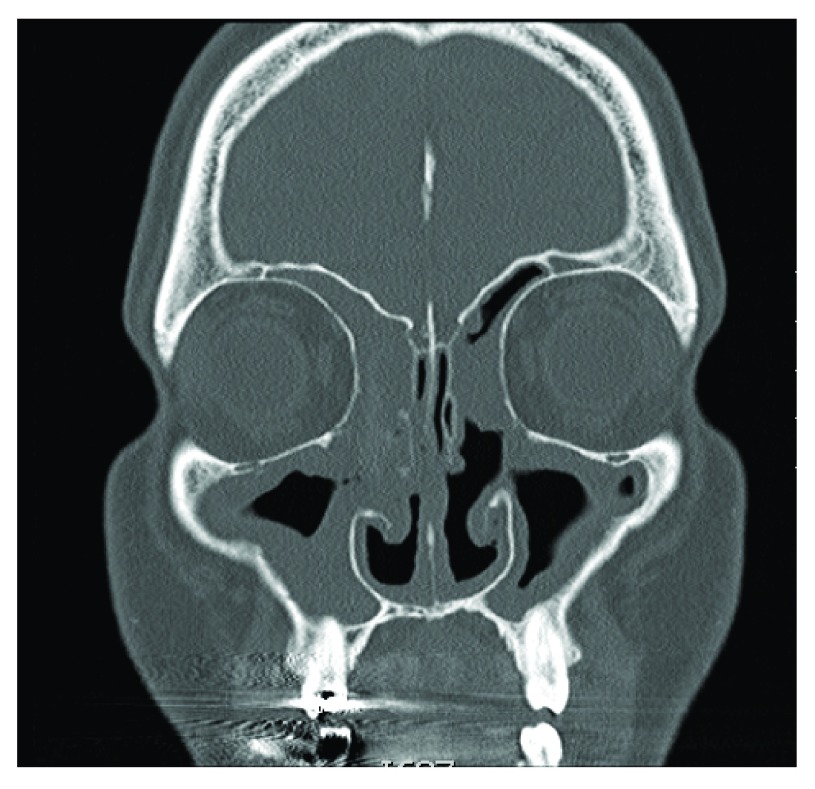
Chronic rhinosinusitis with nasal polyps (CRSwNP). Coronal CT scan of CRSwNP showing extensive polyposis lining the bilateral maxillary, ethmoid, and frontal sinuses.
